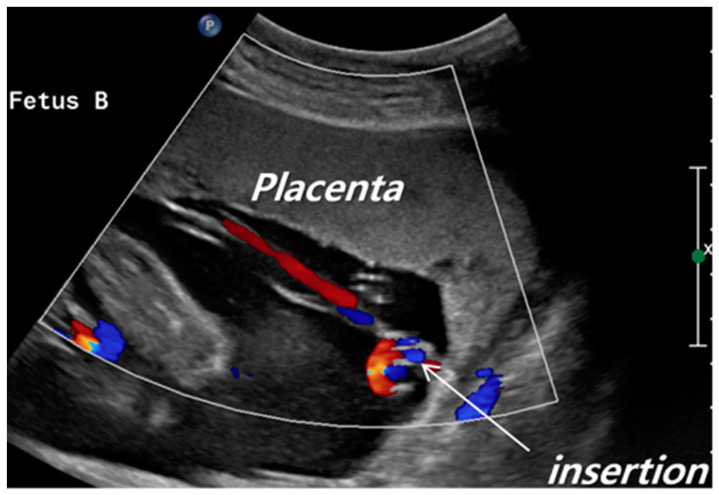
Velamentous cord insertion demonstrated with color Doppler imaging in the mid-trimester.
